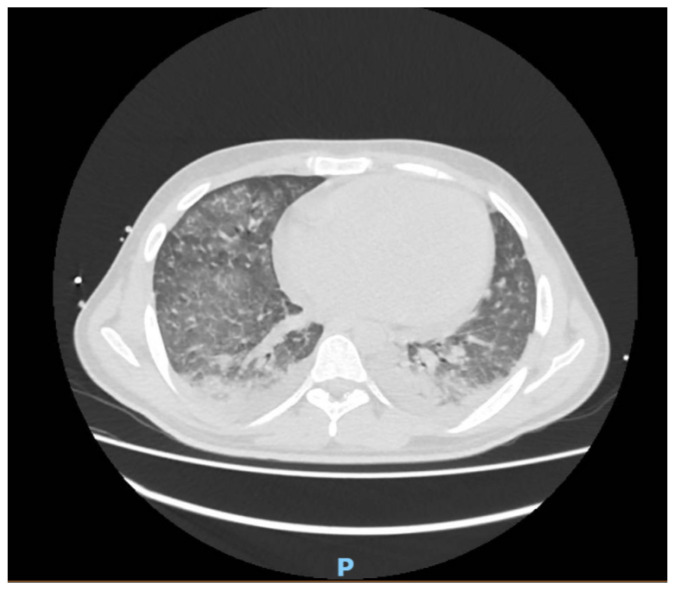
Evidence of widespread ground glass, perihiliar/interscissural fluid thickening, parenchymal atelectasis and pleural effusion at CT, as described in the text.
